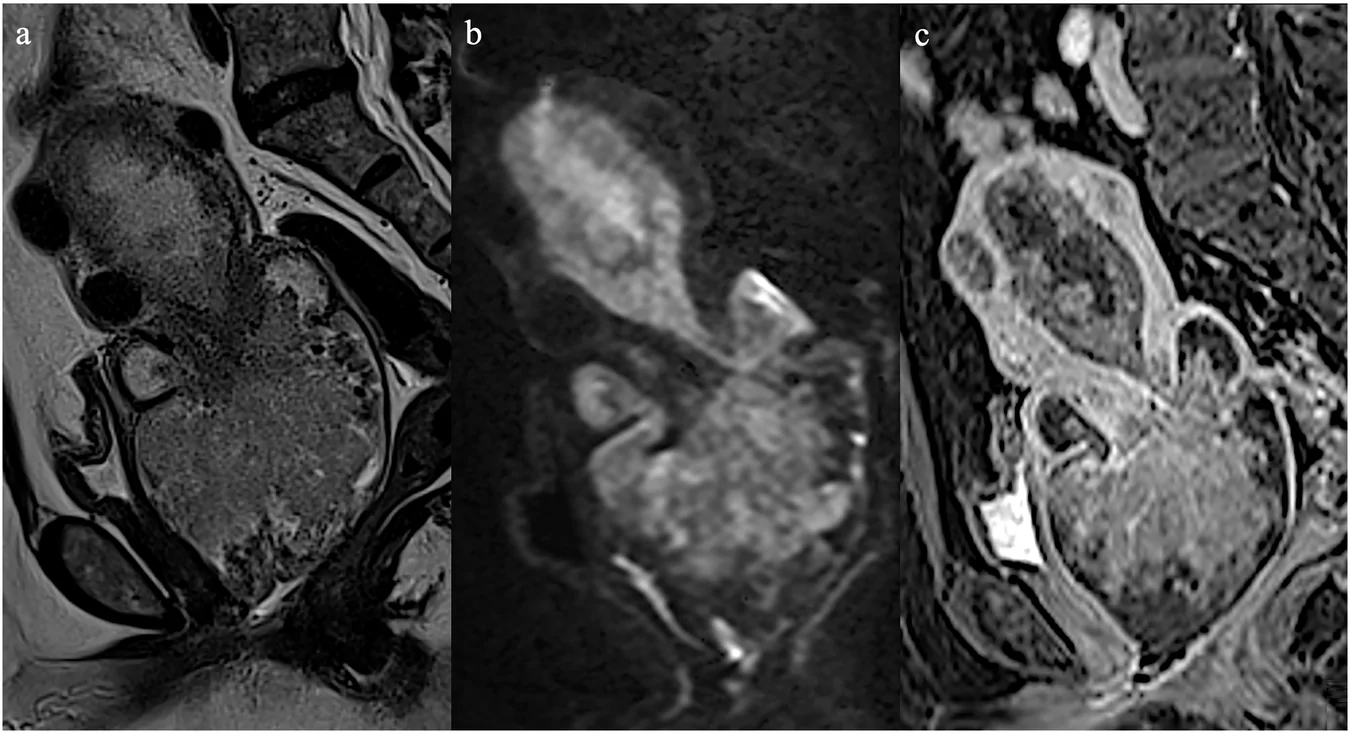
Endometrial carcinosarcoma. a Sagittal T2-weighted sequence; b sagittal DW sequence; c postcontrast fat-saturated T1-weighted sequences. High-grade endometrial carcinosarcoma distending the endometrial cavity with typical extension to the cervix, which is extended without true stromal invasion
